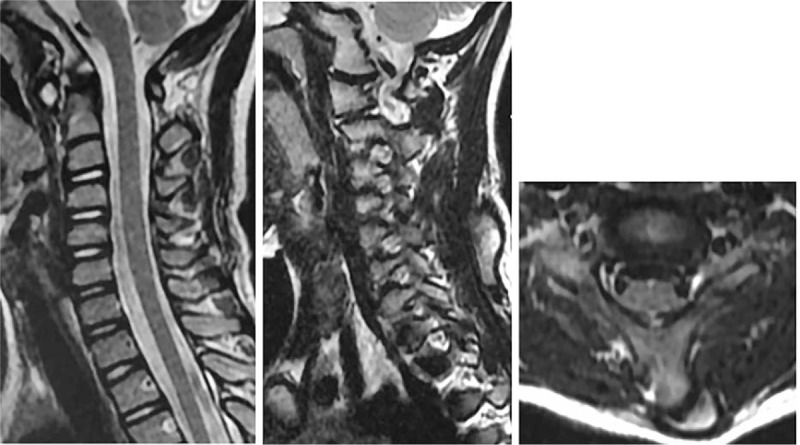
MRI scans showed normal spinal cord and intervertebral discs. MRI also revealed a well-circumscribed cortical bone within the dorsal cervical muscles. The abnormal bone was connected to the spinous process of C5 with cartilage tissue. MRI = magnetic resonance imaging.
